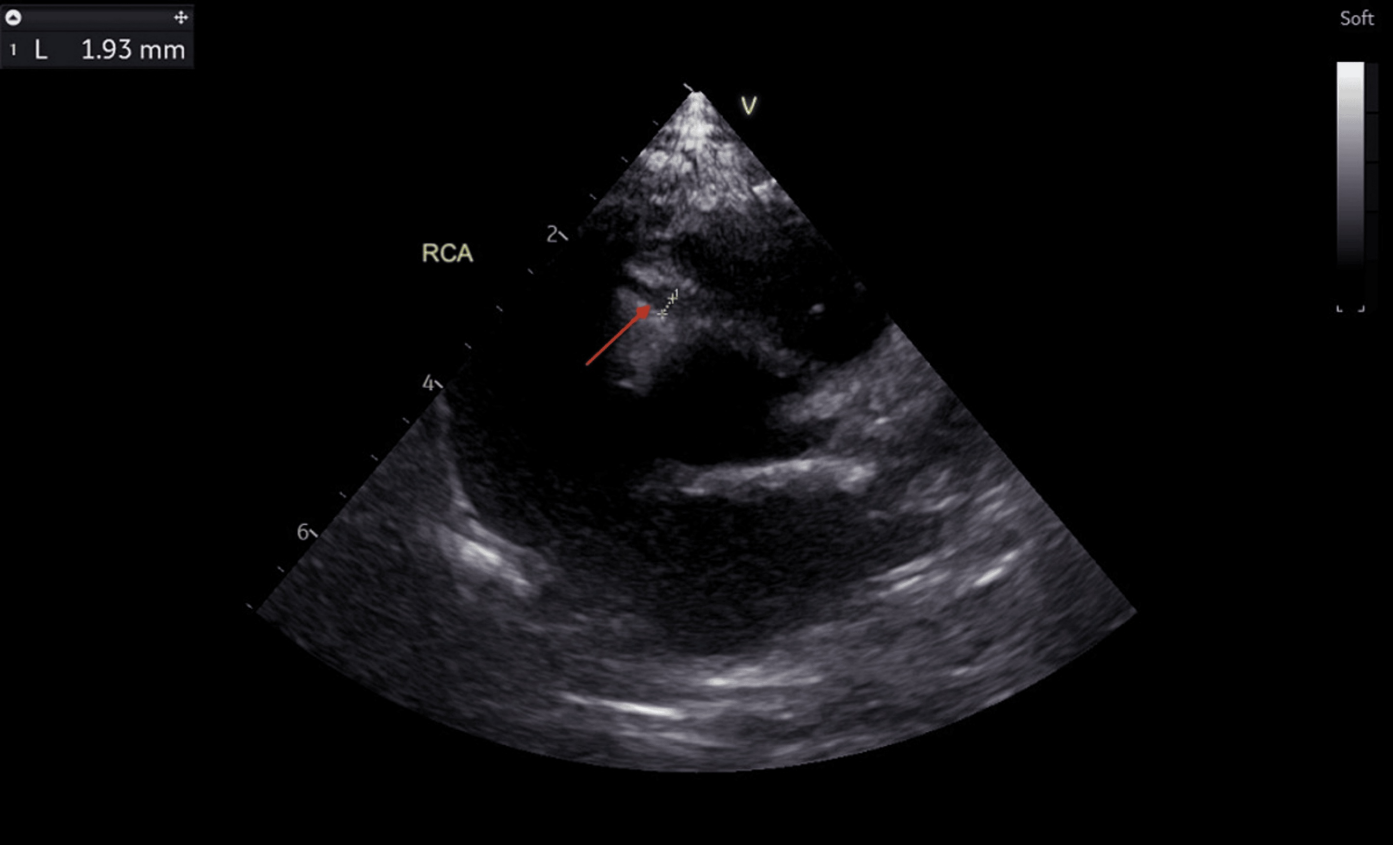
Echocardiogram showing reduction in the right coronary artery dilatation. The red arrow points at the dilated right coronary artery measuring 1.93 mm (Z score: +1.18).
